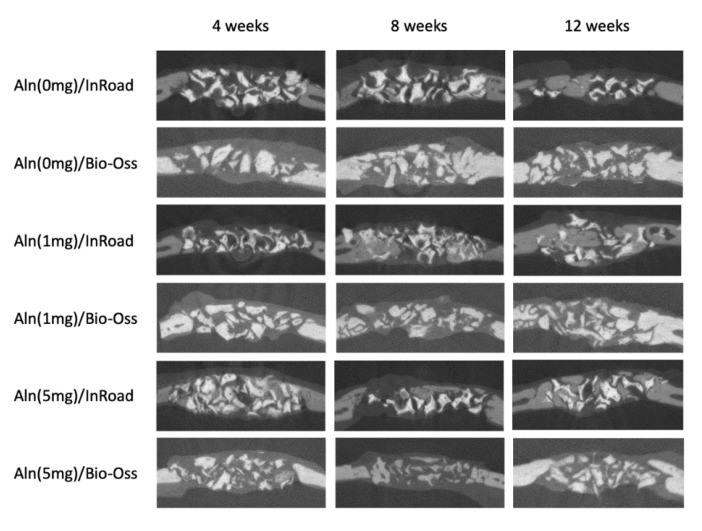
Micro-computed tomography (CT) analysis used to observe the amount of bone formation at the 4, 8, and 12 weeks after implantation. The amount of bone formation was observed using the calculated bone mineral density and bone formation volume (%BV). For the defect area, all tissues of the newly formed bone were included.
